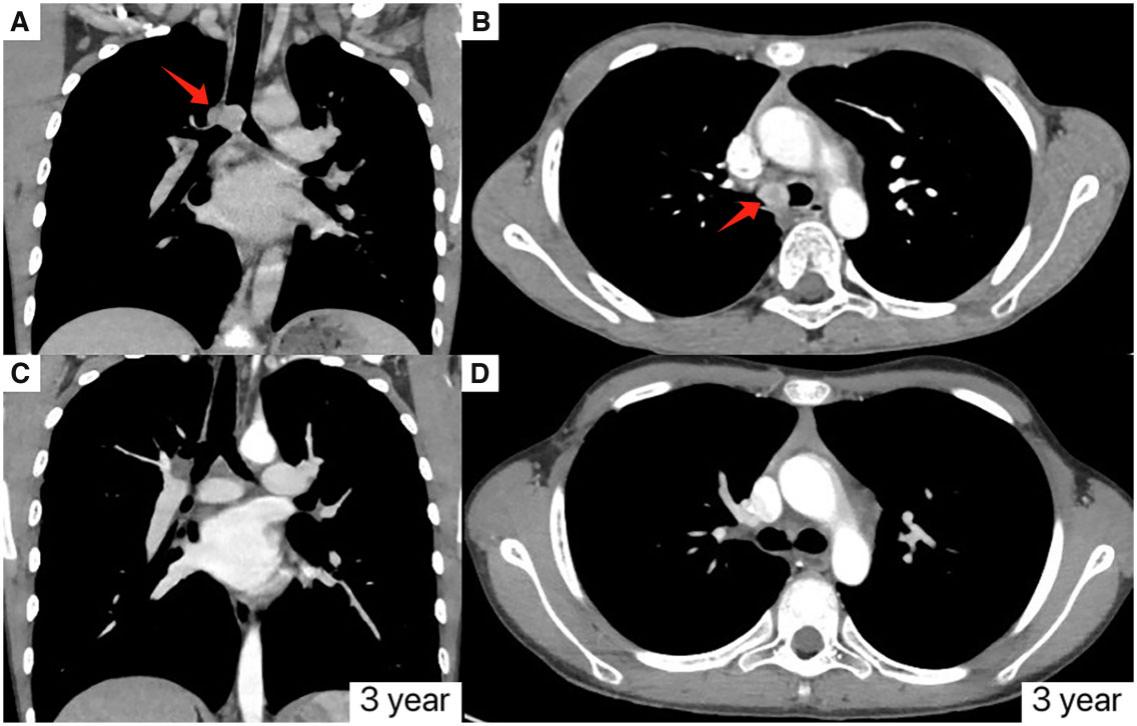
Computed tomographic image of case number 3 in this study. (A) Preoperative computed tomographic image (coronal view). (B) Preoperative computed tomographic image (axial view). (C) Postoperative computed tomographic image (coronal view). (D) Postoperative computed tomographic image (axial view).
